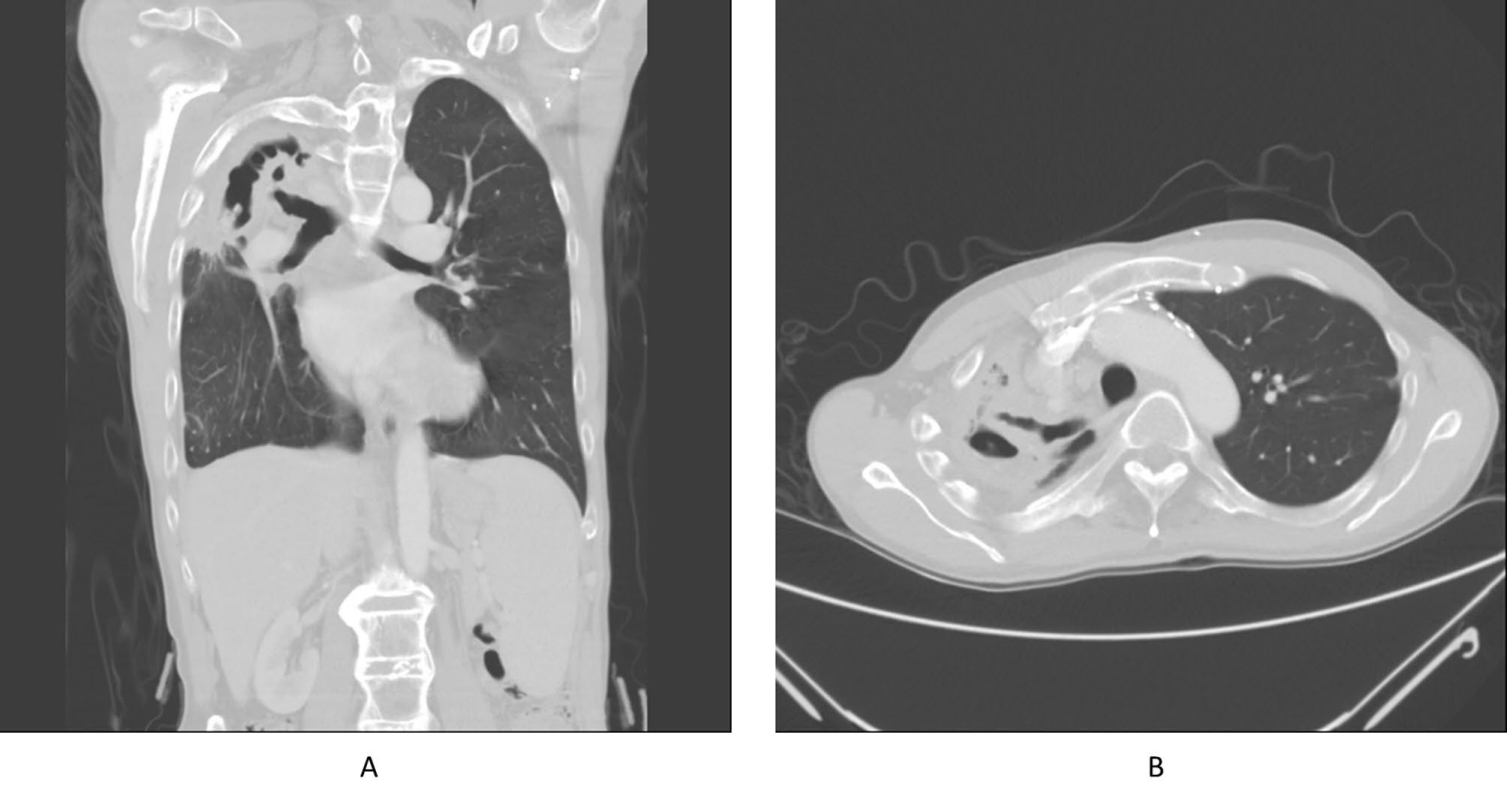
A-B: Computed tomography (CT) scan of patient : The CT scan reveals a large consolidation in the right upper lobe with some calcification observed around the bronchial region, particularly in the upper lobe bronchus
